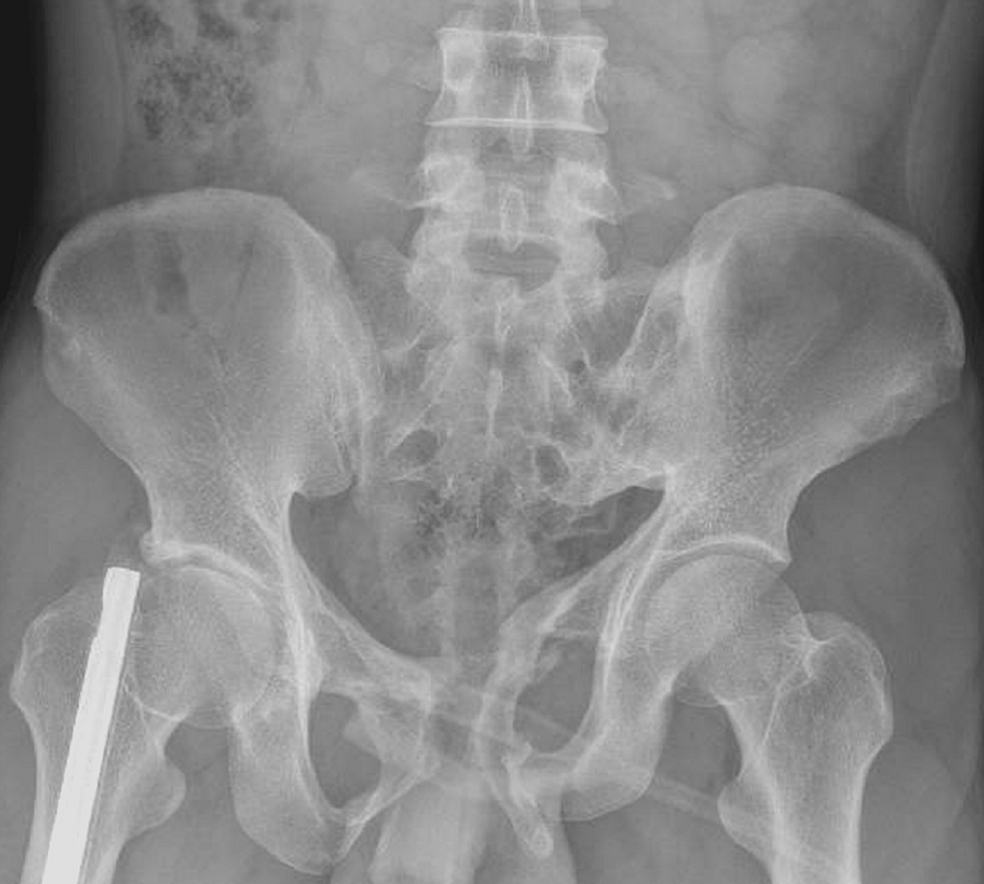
X-ray image of the pelvis The image shows the pelvic fractures and the right femur nail.
